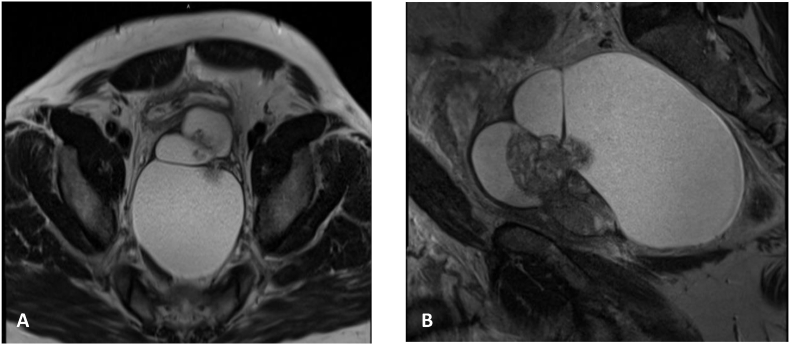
Initial MRI showing large seminal vesicle cyst on axial (A) and sagittal (B) sections.
